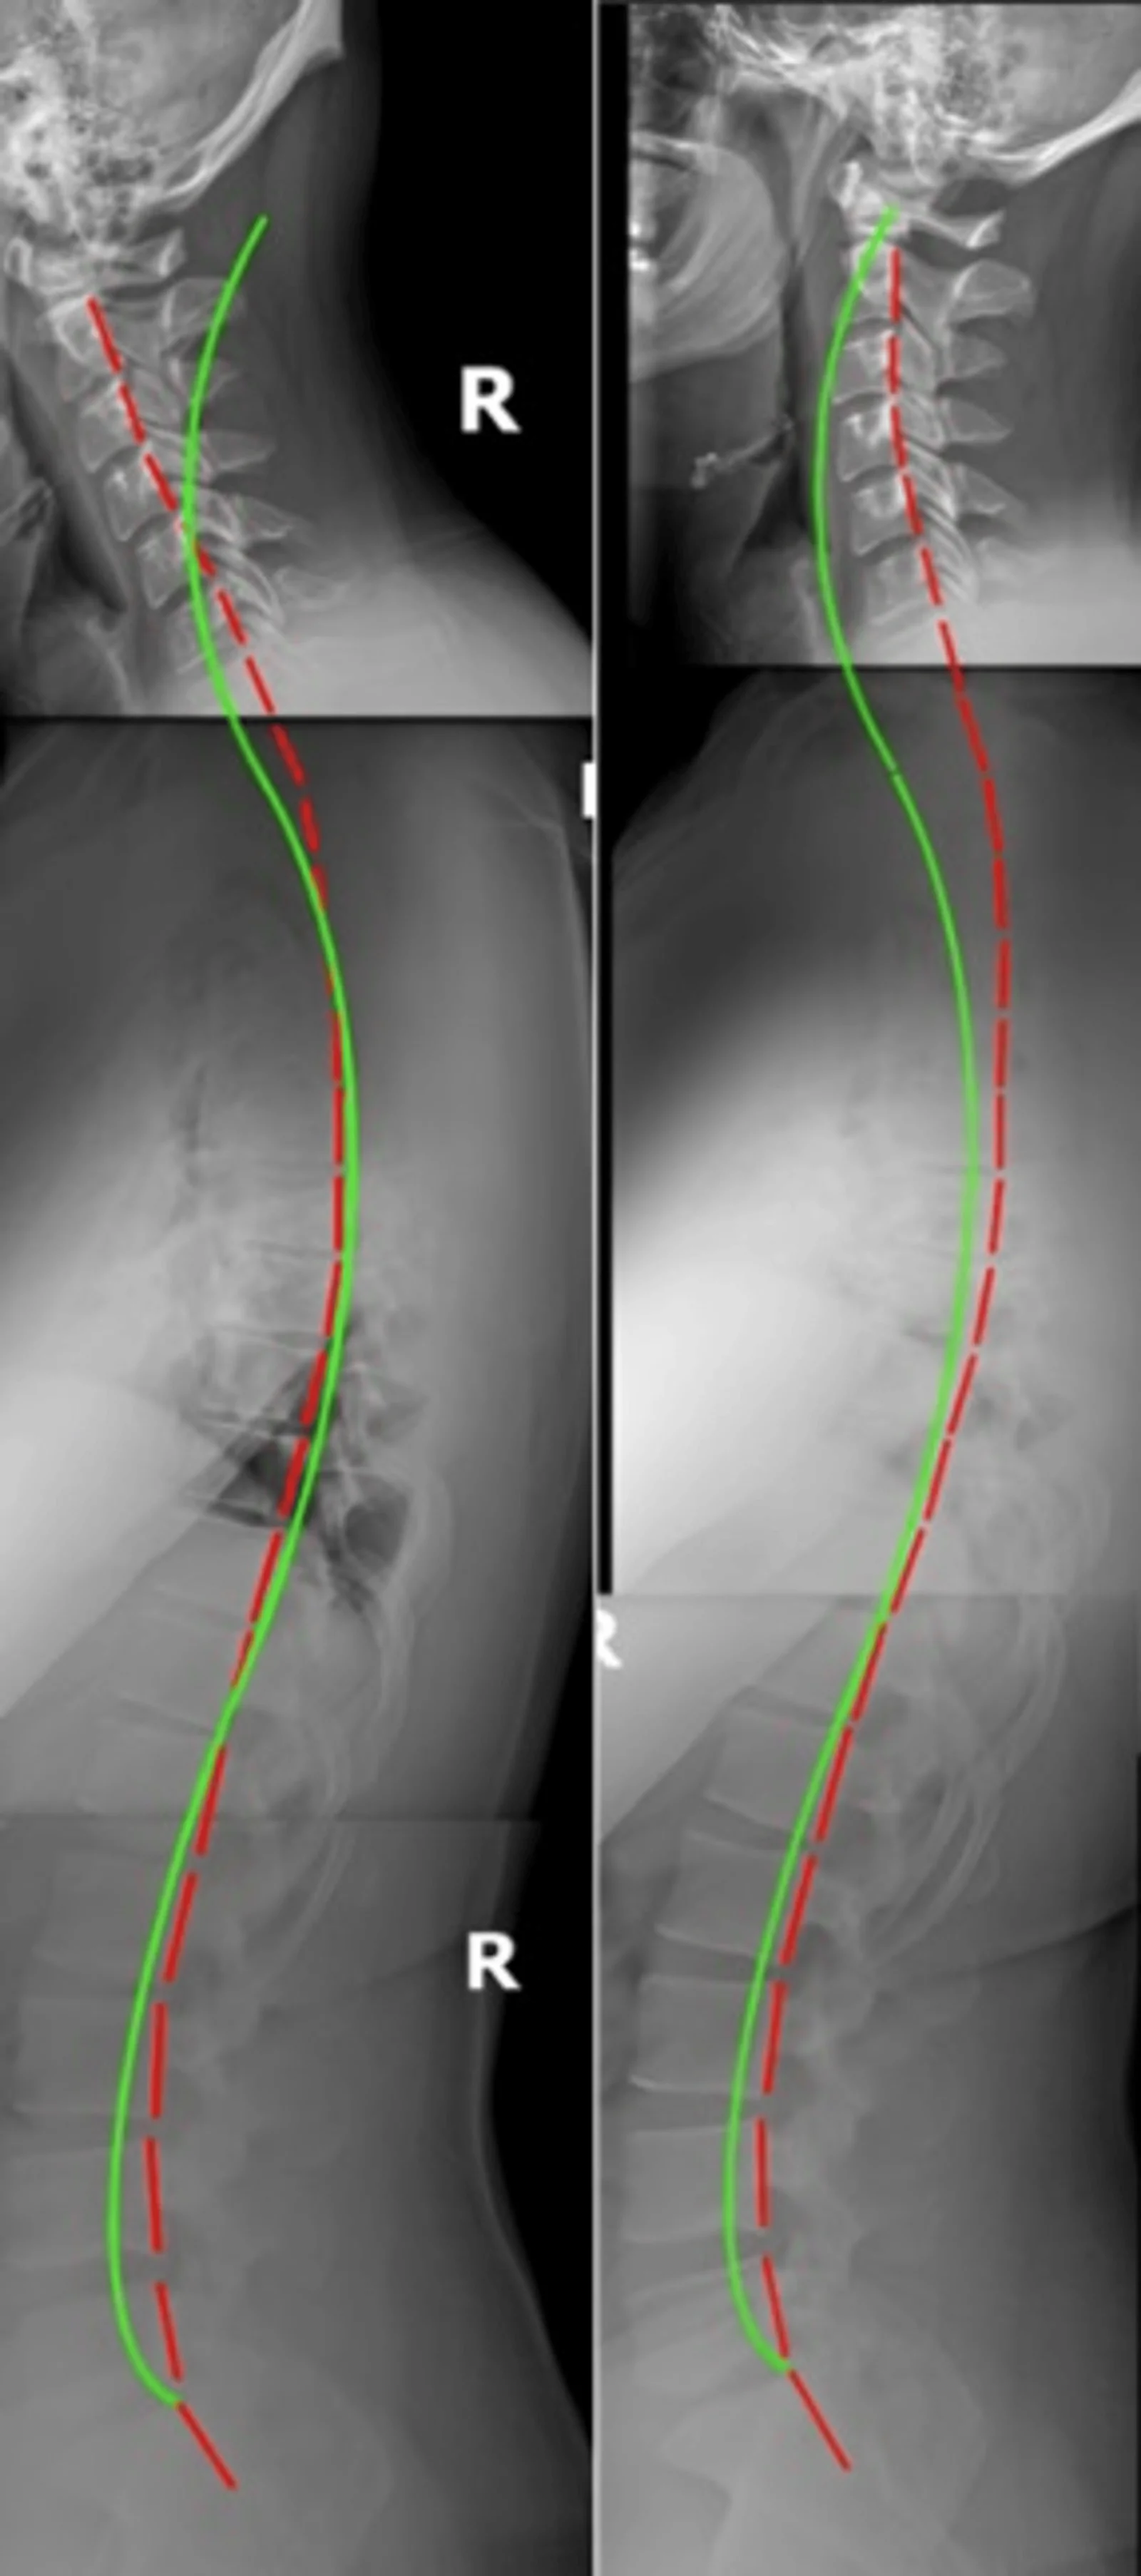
Lateral full spine radiographs: (left) pre-treatment and (right) follow-up.
